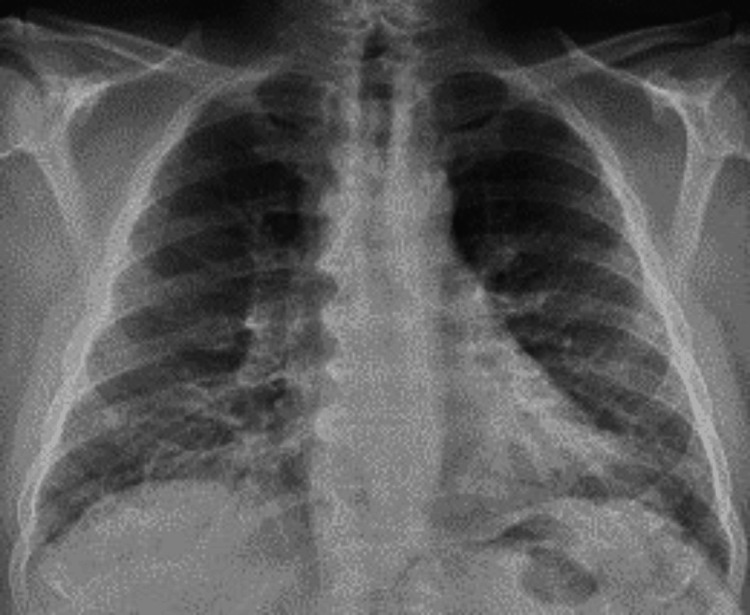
Chest X-ray showing patchy infiltrates in bilateral lower lobes.
